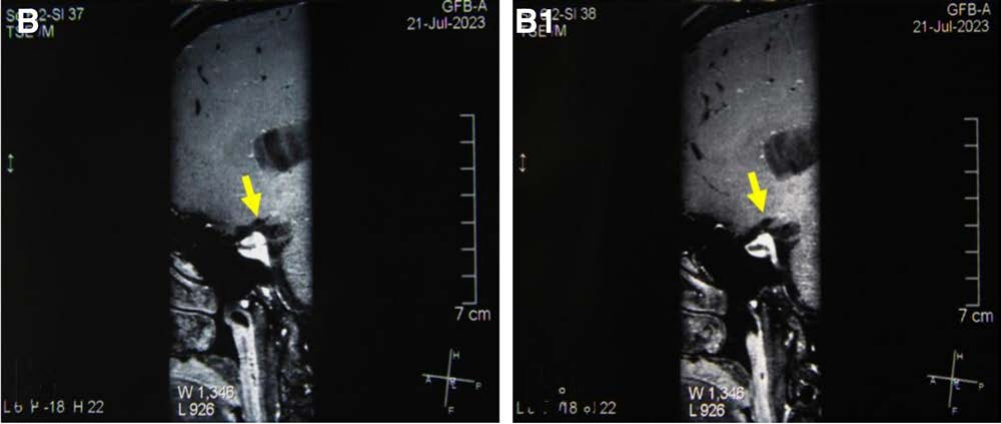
(A and B) HR-MRI was rechecked this year and showed that there was no stenosis in the lumen of the left MCA-M1 segment, and disappearance of the plaque in the M1 segment. (Note: Tiantan Hospital of Beijing changed its examination equipment later, so the magnification was different.)
